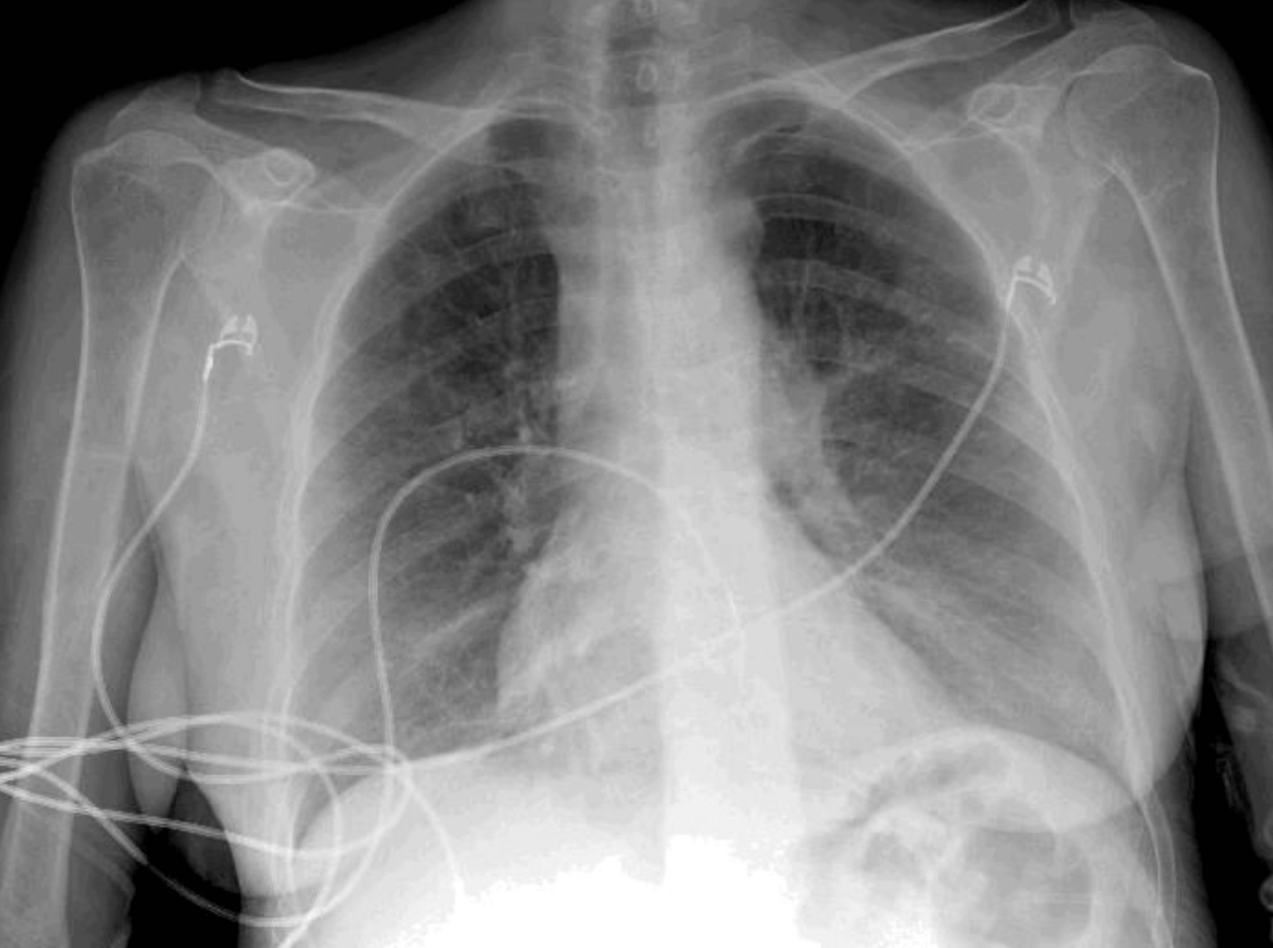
Post-bronchoscopy chest x-ray demonstrating no evidence of infiltrates or complications.
